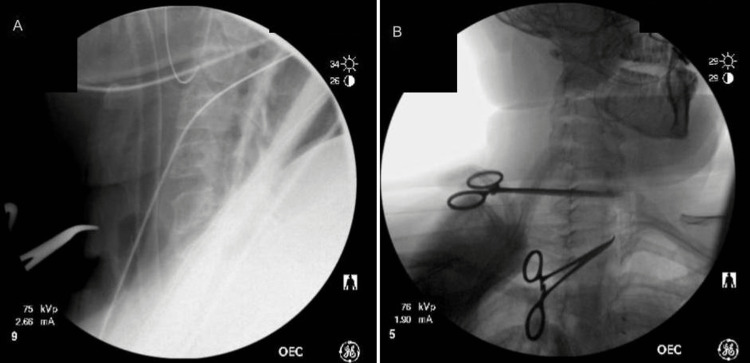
Intraoperative X-ray with lateral (A) and frontal (B) views showing empty right parapharyngeal space.
